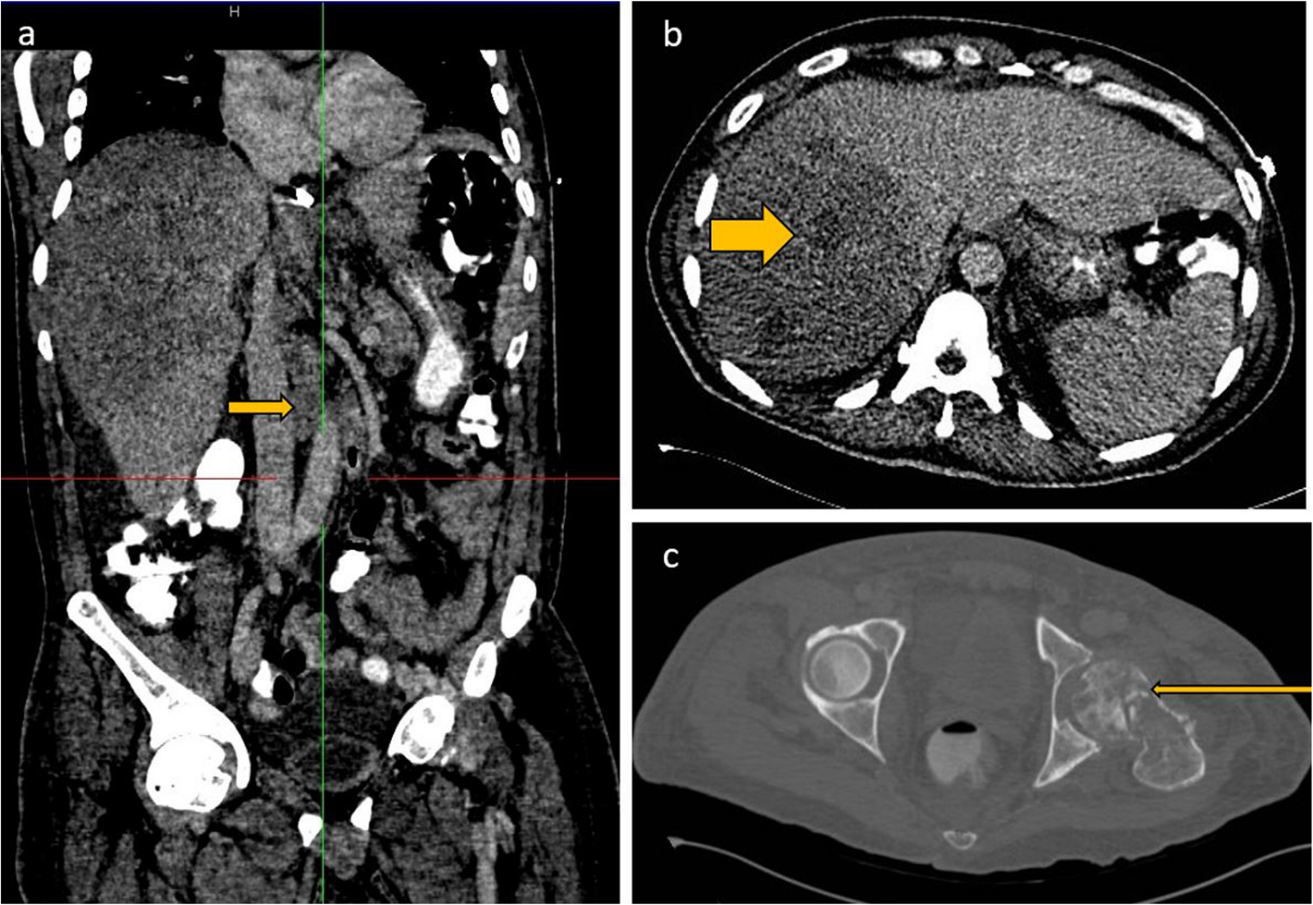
Coronal and axial CECT images of the abdomen (a and b) show hepatomegaly and a heterogenous hypoattenuating mass infiltrating through most of the right lobe of the liver. The infiltrative liver mass had more focal hypoattenuating areas (fat arrow). Aortocaval lymph nodes are also noted (short arrow). Axial CT through the pelvis in bone window (c) shows a permeative process involving the left femoral head and neck and associated pathological fracture
